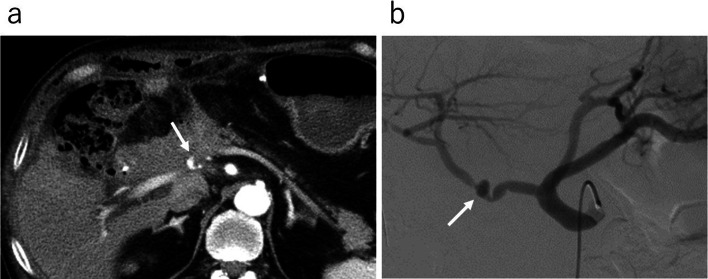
Image findings of pseudoaneurysm after PD. a Contrast-enhanced CT showing the formation of a pseudoaneurysm at the end of the GDA (arrow →). There was widespread hematoma in the surrounding area. b Angiography showing a pseudoaneurysm at the end of the GDA (arrow →)
